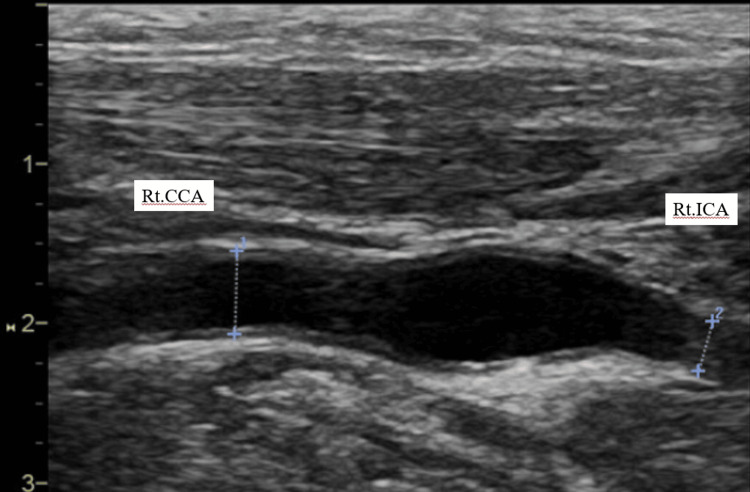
Carotid ultrasonography of the right (Rt.) common carotid artery (CCA), bifurcation, and internal carotid artery (ICA, long-axis view) Ultrasonography demonstrates an adventitia-to-adventitia diameter of 5.2 mm in the Rt. CC and 3.2 mm in the ICA, yielding a ratio of 0.61. This vascular morphology resembles the characteristic “champagne bottle neck” sign.
